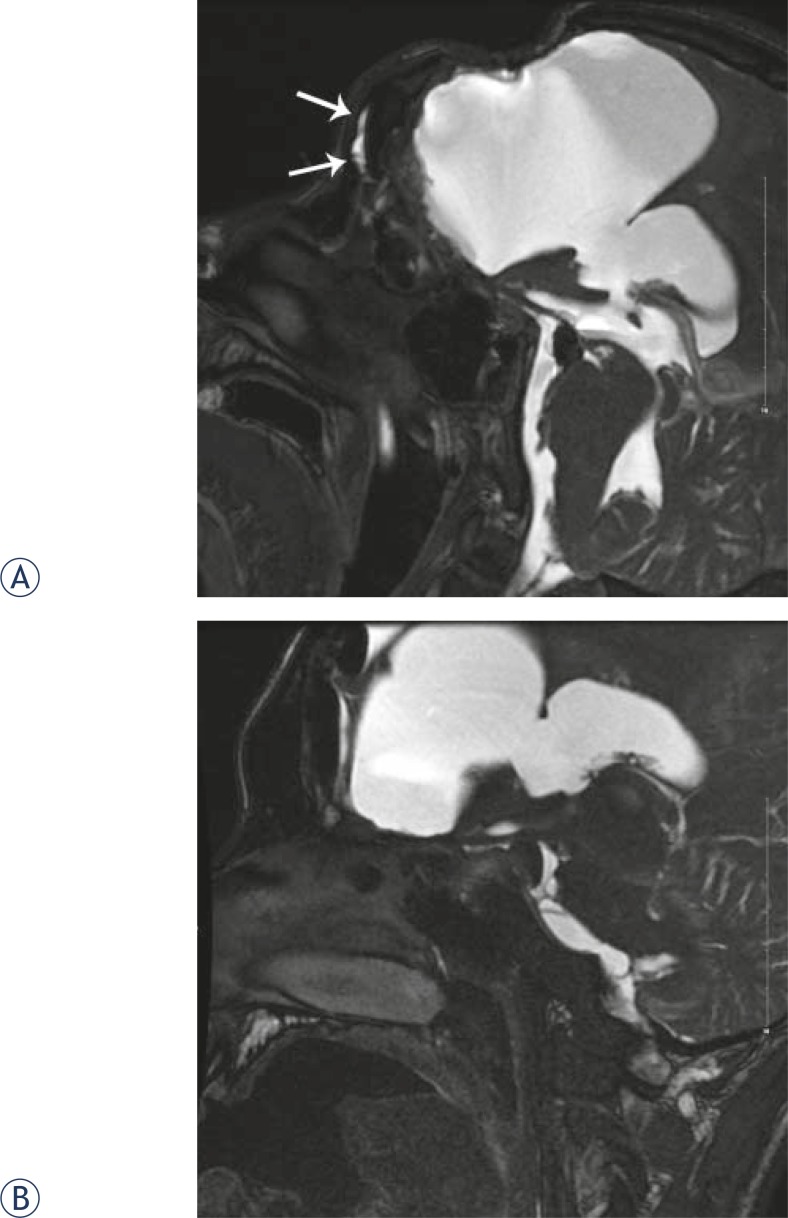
A male patient aged 21 years with severe craniocerebral injury. Surgery was performed to remove part of the frontal bone and contused brain tissue of frontal lobe. One week after surgery, cerebrospinal fluid rhinorrhea was present in left nose. CT scan showed multiple basilar skull fracture and failure in ethmoid sinus repair. MR cisternography showed that high-signal liquid was present in frontal sinus in the prone position (3A) but not in the supine position (3B) in T2-weighted image, thus cerebrospinal leak in frontal sinus was diagnosed. It was observed during surgery that the crack fracture was present in the posterior side of frontal sinus with damaged *dura mater*, which communicated with nasal cavity, and rhinorrhea disappeared after the surgery.
